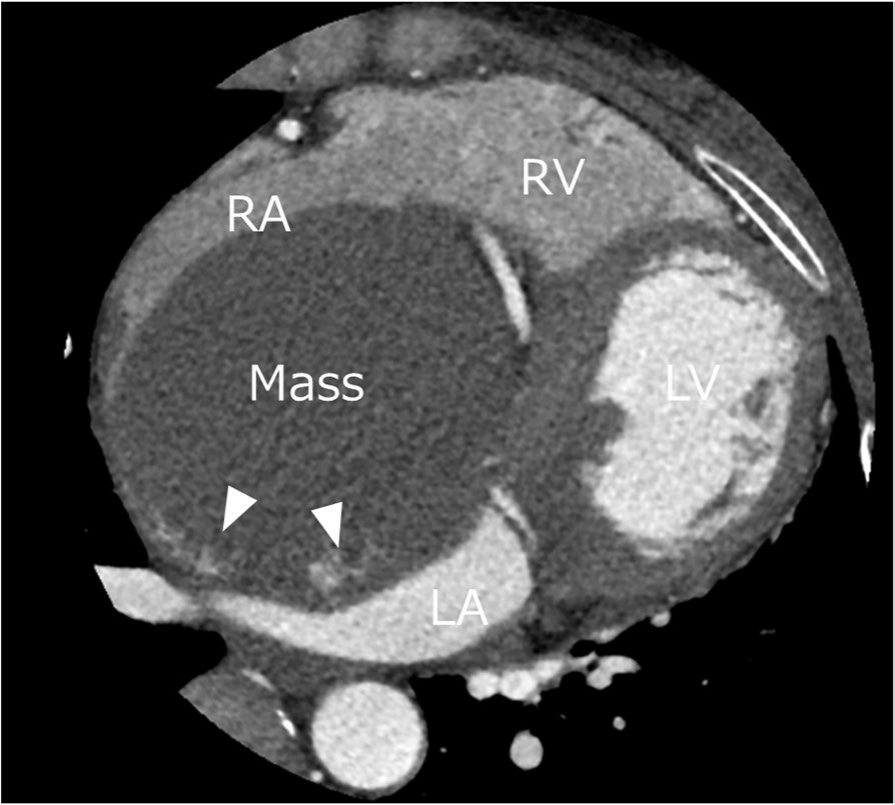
Contrast-enhanced computed tomography. The right atrial chamber is occupied by the mass having partial contrast enhancement, which suggests the possibility of feeding arteries. LA, left atrium; LV, left ventricle; RA, right atrium; RV, right ventricle
